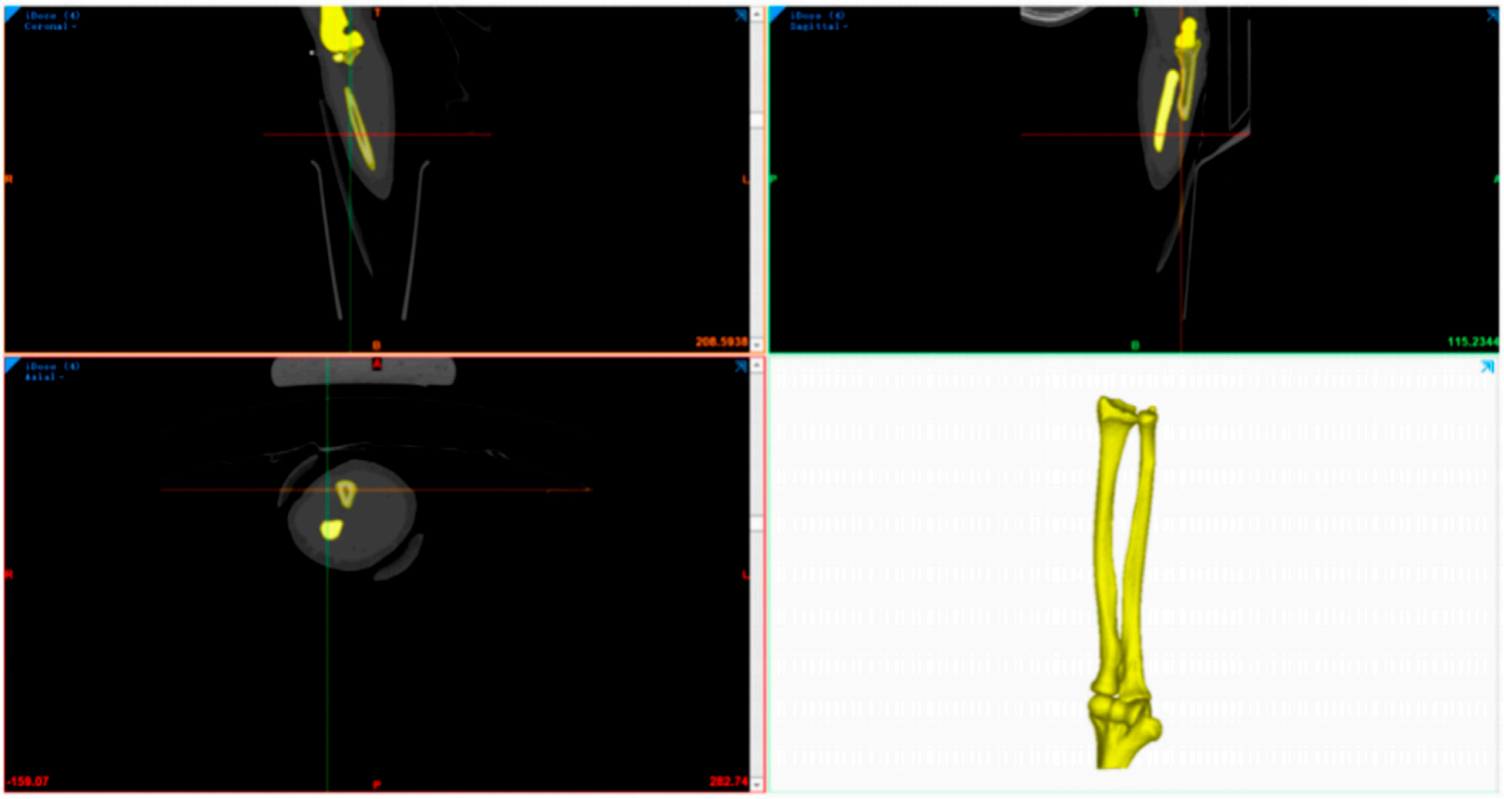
Three–dimensional reconstruction bone map of the CT wrist joint..
